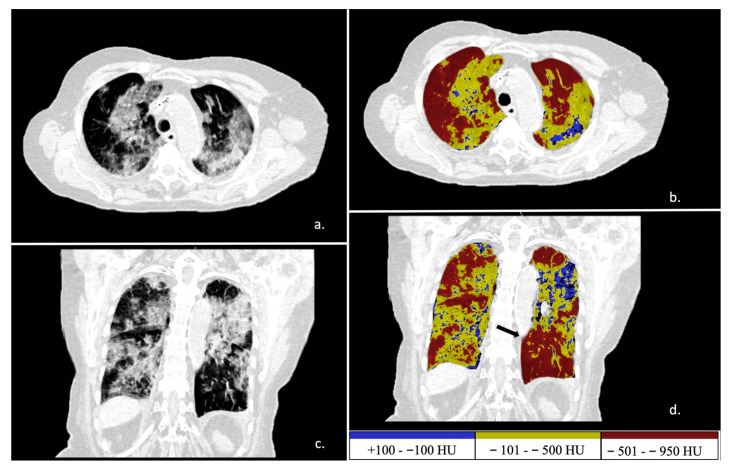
Automatic tissue density analysis. In images (a,b), axial views. In images (c,d), coronal views. In images (a,c), unenhanced CT slices of an extensive lung COVID-19 involvement with bilateral infiltration. In images (b,d), the software-dedicated analysis differentiated non-aerated (blue) and poorly aerated (yellow) tissue. In red, well-aerated lung tissue. In image (d), the black arrow indicates the exclusion of Hilary structure from high-density tissue. Color bar values on the bottom right.
